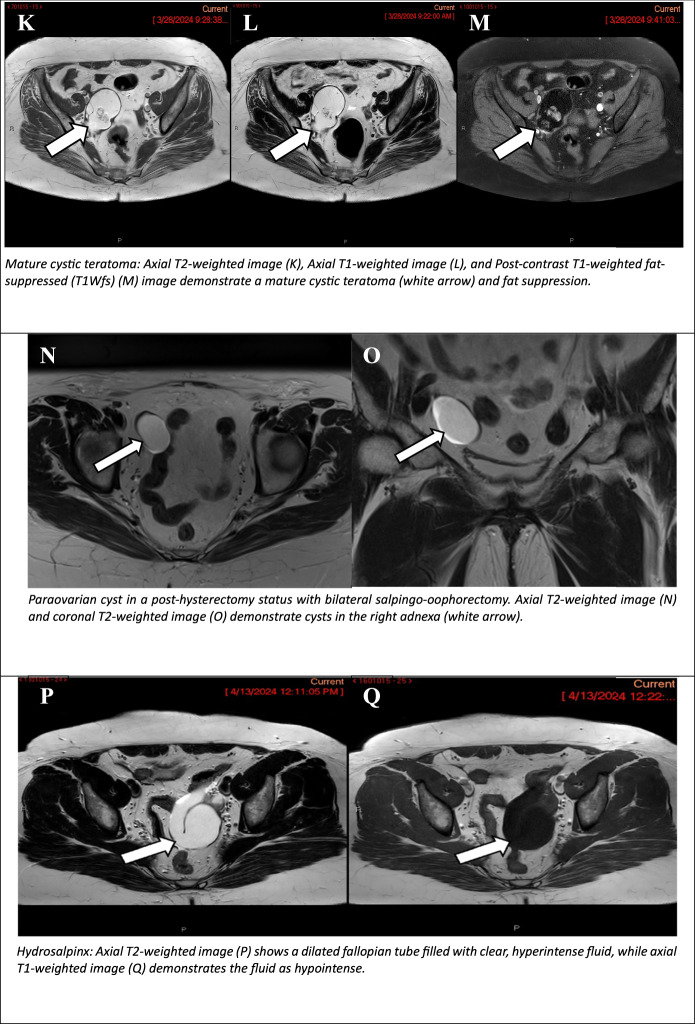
O-RADS MRI 2 (non-physiological lesions): adnexal lesions are almost certainly benign. Unilocular cystic lesion measuring approximately 4 cm. Axial T2WI (A) shows a hyperintense lesion, while coronal T2WI (B) confirms its location. Axial T1-weighted image (C) demonstrates hypointense follicles of the right ovary, and DIXON image (D) provides additional characterisation of the lesion (white arrows). Endometrioma: axial T2-weighted (E) image showing the “black dot sign” indicative of chronic haemorrhage (white arrow). Axial T1-weighted fat-suppressed (T1Wfs) (F) image demonstrating the “shading sign” (white arrow), which represents recurrent haemorrhage. Axial T1-weighted image (G) confirms high SI of the cystic content (white arrow). Ovarian cystadenofibroma: Axial T2WI shows a dark spot (H, I orange arrow) and thick septations within the mass. No diffusion restriction on high b-value DWI and ADC map (J). Mature cystic teratoma: Axial T2WI (K), Axial T1-weighted image (L), and Post-contrast T1-weighted fat-suppressed (T1Wfs) (M) image demonstrate a mature cystic teratoma (white arrow) and fat suppression. Paraovarian cyst in a post-hysterectomy status with bilateral salpingo-oophorectomy. Axial T2WI (N) and coronal T2WI (O) demonstrate cysts in the right adnexa (white arrow). Hydrosalpinx: axial T2WI (P) shows a dilated fallopian tube filled with clear, hyperintense fluid, while axial T1-weighted image (Q) demonstrates the fluid as hypointense
